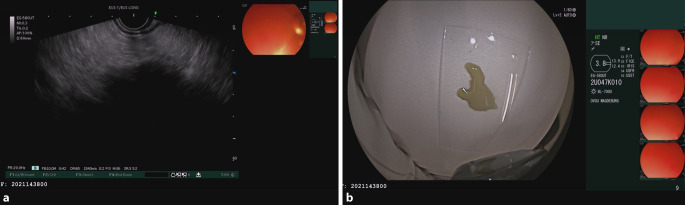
EUS-geführte Punktion: a Pankreas unauffällig, wie vorab bereits in der CT zu erkennen – ein semiliquides Areal im Isthmusbereich. b Punktat nach transgastraler Punktion mit 19-G-Nadel → Aspiration von gelbgrauer Flüssigkeit – alte Nekrose nach traumatischer Pankreatitis
